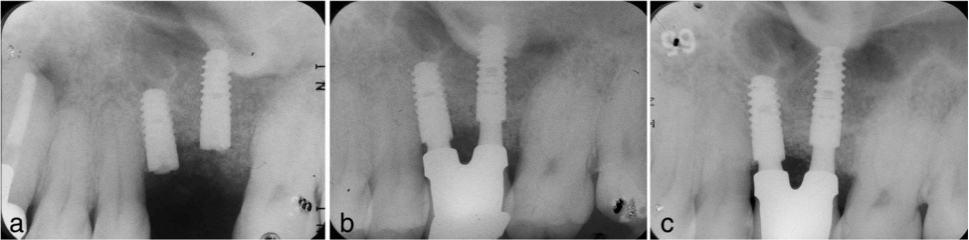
Case 2. Periapical X-ray films showing progression of intraimplant bone formation from implant date to 3 years and 8 months after procedure. a June 2008. b November 2008. c February 2012
